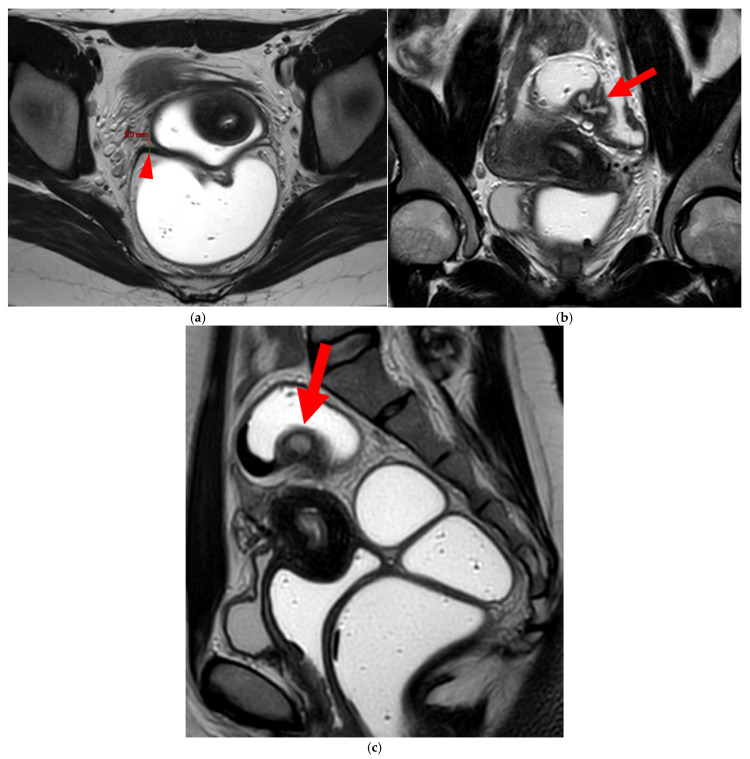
Pelvic MRI scan of a patient with HTD type 6 USLs. (a) Axial T2WI shows a thick (3 mm) right USL (*arrowhead*) with regular margins, initially classified as a type 3A USL; the left USL is not visible (type 1). (b,c) Coronal (b) and sagittal (c) T2WI show sigmoid colon wall infiltration appearing as a “medallion-shaped” lesion (*arrows*). The presence of “visceral” involvement of the digestive tract leads to the reclassification of these USLs as type 6.
